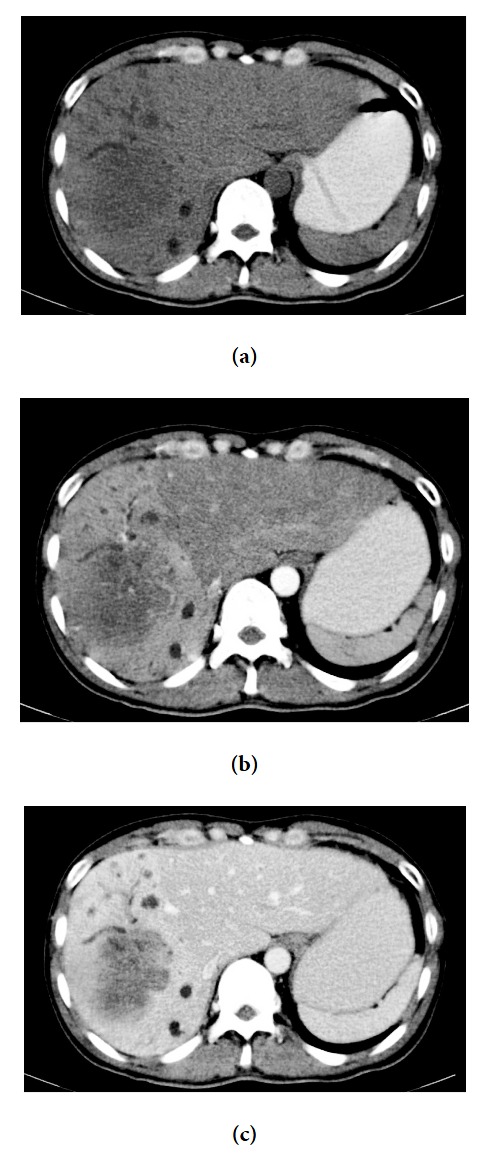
Computed tomographic scan showed tumor (a) hypoattenuating in precontrast phase, (b) no enhancement in arterial phase, and (c) mild enhancement in portal venous phase.
